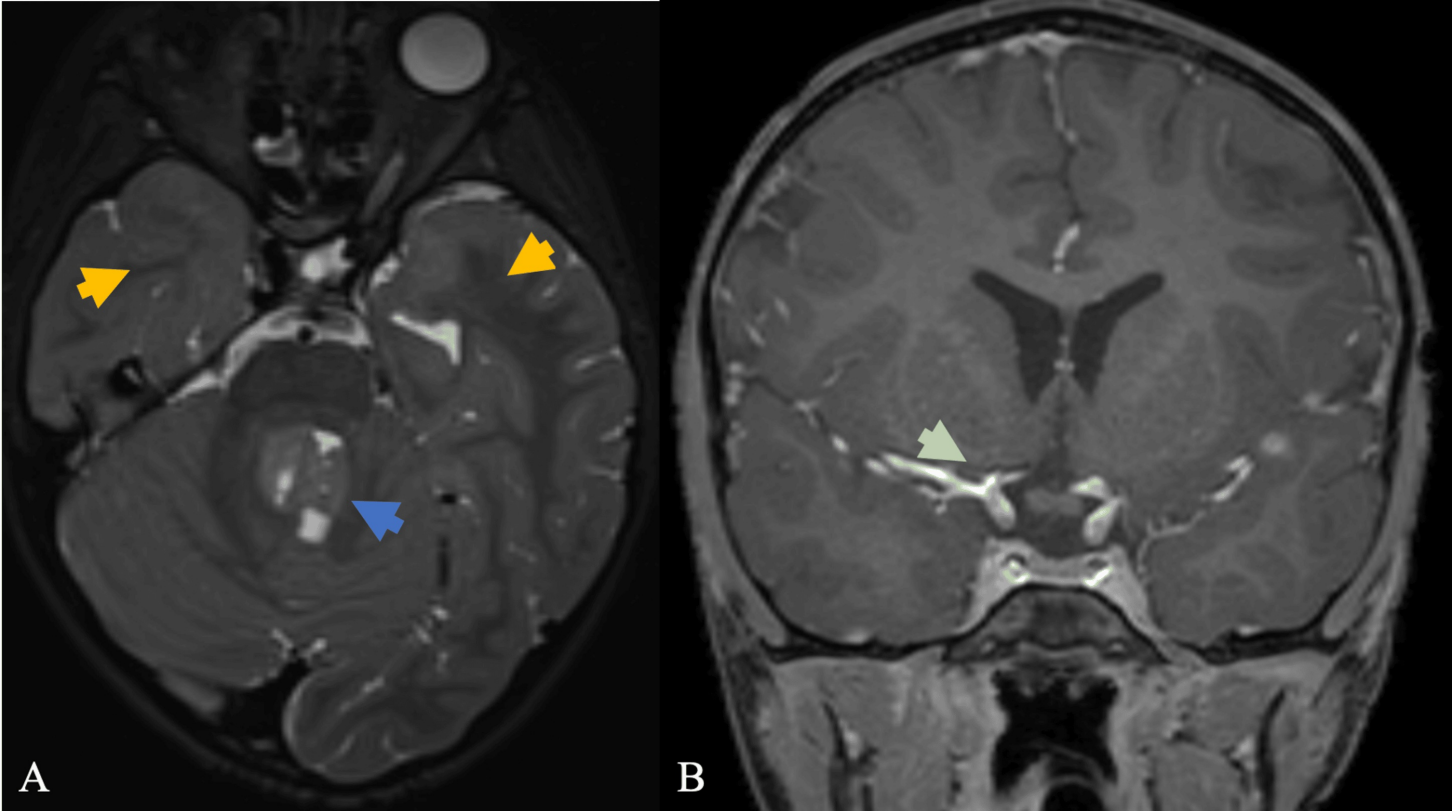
(A) Pre-radiation axial T2-weighted MRI through the skull base showing a T2 hyperintense mass in the fourth ventricle (blue arrow), and no abnormal signal is demonstrated in the anterior temporal lobe white matter (yellow arrow). (B) Immediate post-radiation coronal gadolinium-enhanced T1-weighted MRI through the anterior circulation showed a normal caliber M1 segment of the right MCA (green arrow) MRA: middle cerebral artery
